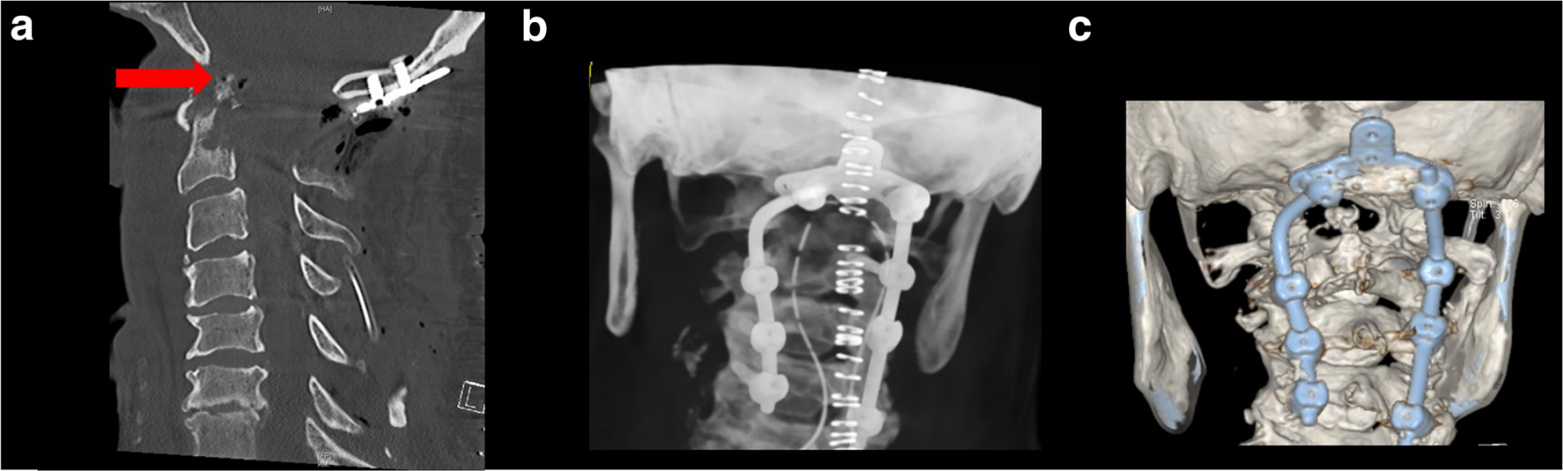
Postoperative CT scan of cervical spine (a) and different 3-dimensional reconstruction (b,c) revealed a regular placement of screws and air in the OP from biopsy (red arrow)
